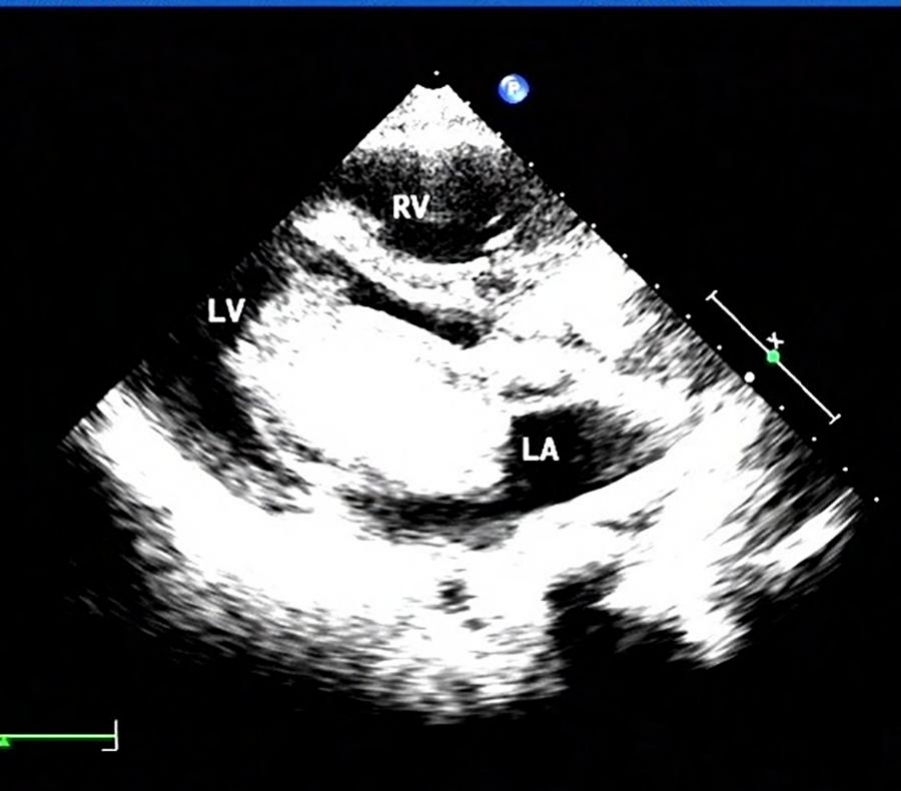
Transthoracic echocardiogram (apical four-chamber view) showing a large, hyperechoic atrial myxoma (arrow) attached to the interatrial septum.
